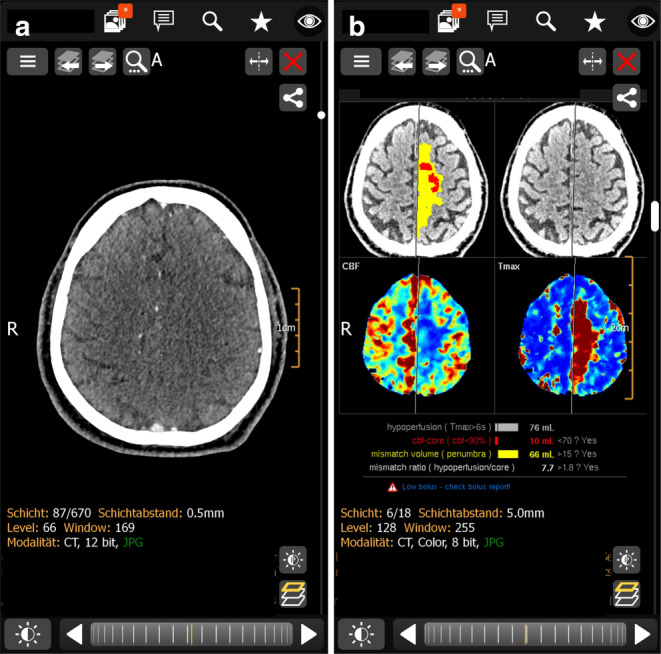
A 50-year-old man with right-sided hemiplegia and nonfluent aphasia since 1h was admitted in the evening. NIHSS was 17. The left-sided anterior cerebral artery occlusion was difficult to see on CT-Angiography (a, *left image*) using a mobile phone, but readily displayed on CT-Perfusion maps and the corresponding hypoperfusion- and core segmentation (b, *right image*)
